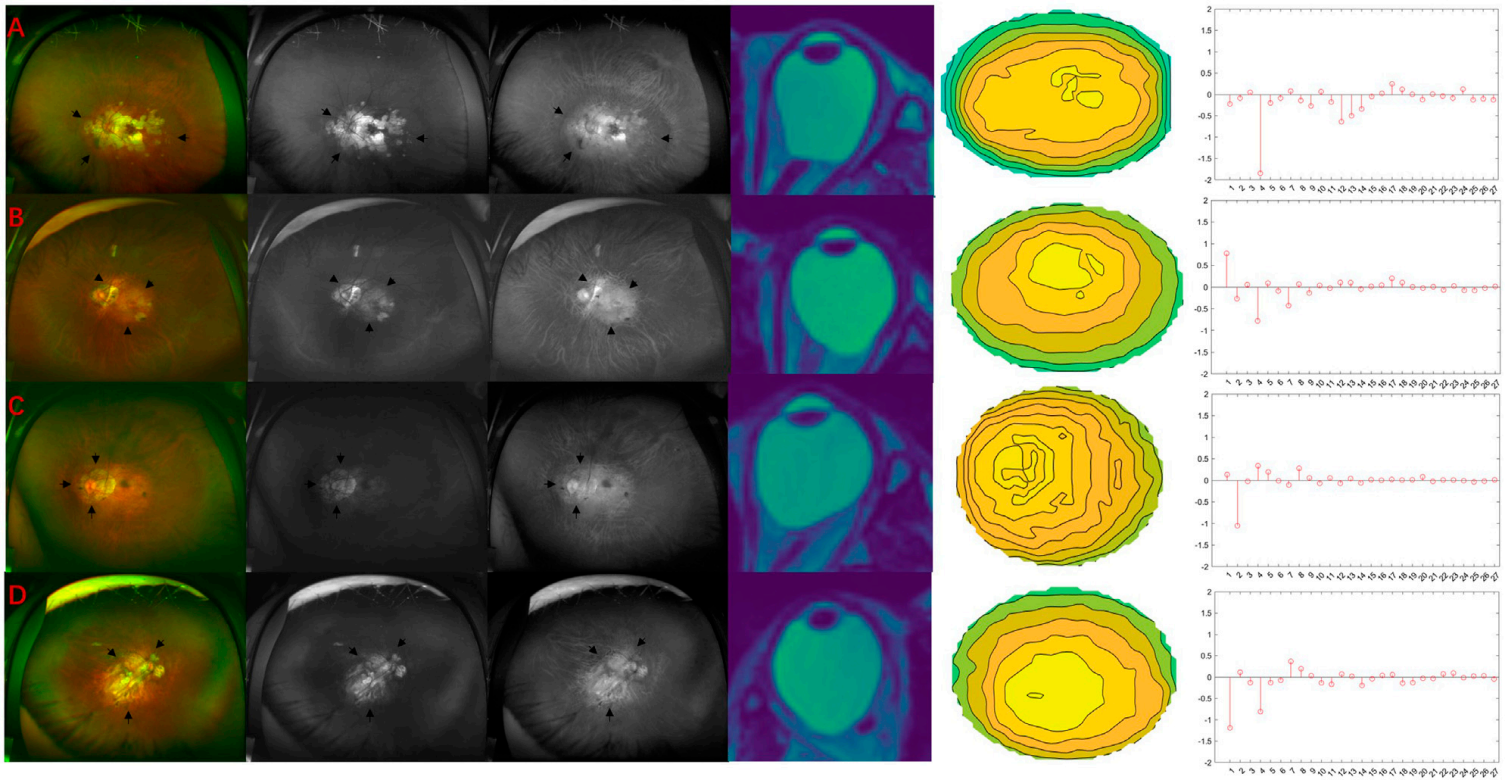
Four different types of PS. Black arrows indicate the borders of the staphyloma. (A) Wide macular PS. The nasal edge of macular PS exists more nasally beyond the nasal edge of the optic disc. 2D MRI tomographic image shows that the eyeball’s posterior part is blunt. The height map of the posterior part of the eyeball shows spare contour lines in the central part. Note spherical aberration (12th) is negative, indicating that the local bulge in the center is not apparent. (B) Narrow macular PS. The macular PS’s nasal edge is along the optic disc’s nasal edge. 2D MRI tomographic images show sharp bulges at the back of the eyeball. The height map of the back of the eyeball shows dense contour lines in the central part. Note the spherical aberration (12th) is positive, indicating that the central part is prominently raised. (C) Peripapillary PS. Areas of marked retinal atrophy are seen around the optic disc. The 2D MRI tomographic image shows that the eyeball protruded significantly in the direction of the optic nerve. The height map of the back of the eyeball shows denser contour lines on the left (nasal side). Note the larger horizontal Tilt (2nd term). (D) Inferior PS. 2D MRI tomographic image shows that the posterior pole of the eyeball is prominent, and there is no significant horizontal tilt. The contour map of the back of the eyeball shows that the prominent position is lower. Note the large vertical tilt (1st term).
